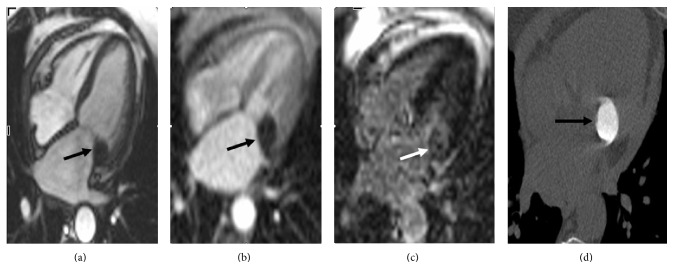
Balanced steady state free precession (SSFP) sequence image (a) shows hypointense lesion with well-defined borders (black arrow). First pass image (b) demonstrates no contrast enhancement. Delayed phase postcontrast phase sensitive inversion recovery (PSIR) image (c) shows peripheral enhancement. Multidetector cardiac CT image for calcium scoring (d) shows diffuse calcific lesion with well-defined borders located in mitral annulus (black arrow).
